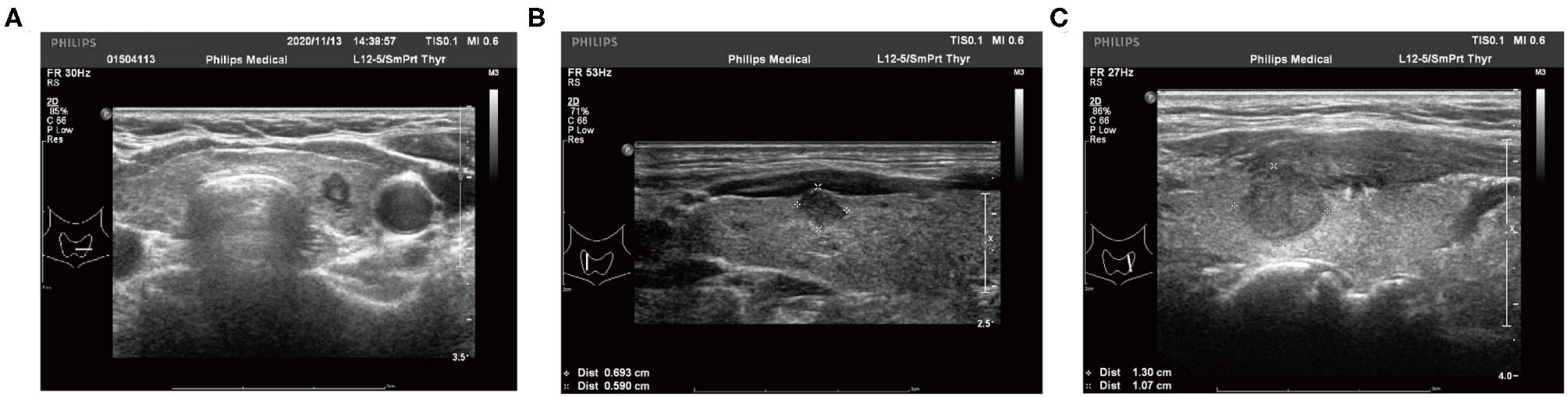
Typical ultrasound imaging of different extrathyroidal extension status. (A) The tumor is totally localized in the thyroid lobe. (B) The tumor invades the capsule of the thyroid lobe. (C) The tumor presents as an extrathyroidal extension.
